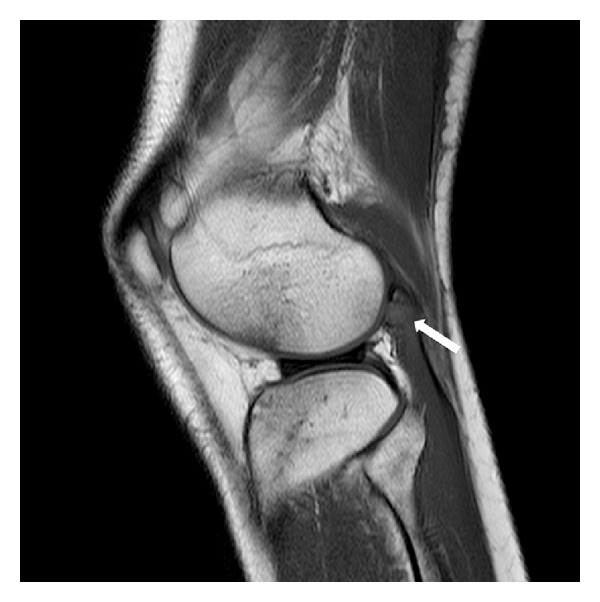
T1-weighted fast spin-echo MRI showed bone contusions in the anterior femoral and tibial regions at the lateral tibiofemoral compartment, related to hyperextension and valgus mechanisms of injury.
